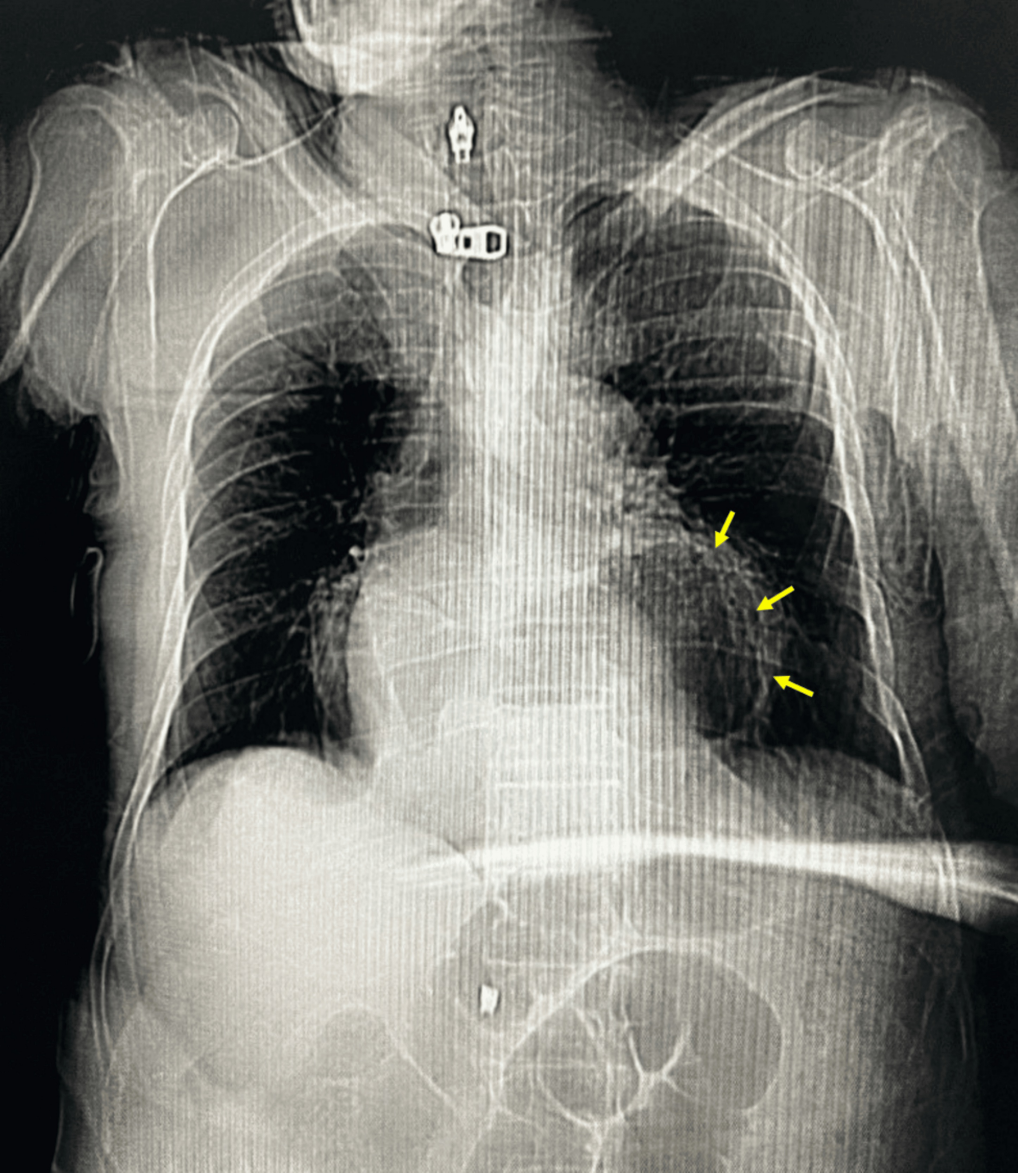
Chest scout radiograph‎ Frontal scout view demonstrating a large retrocardiac air-containing structure (arrows) occupying the left hemithorax, with rightward mediastinal shift.
